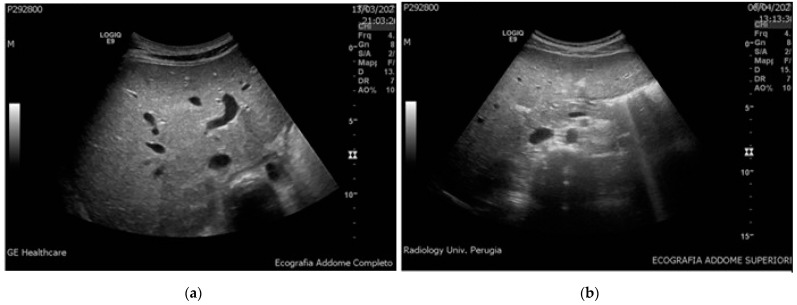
(a) Ultrasonography of the abdomen performed at discharge demonstrating normal dimension of the liver and a homogenous pattern. (b) Ultrasonography of the liver performed during follow-up demonstrating hepatomegaly and a hyperechoic liver, suggestive of hepatic steatosis.
